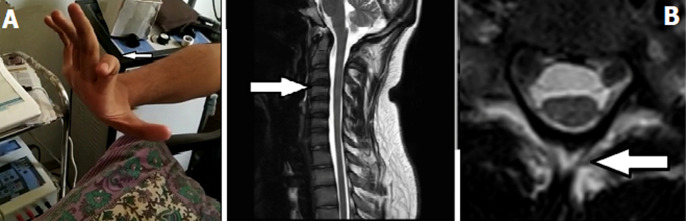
clinical as well as radiological features A) atrophy of the forearm leads to the difficulty of the movements of the hands; B) MRI findings shows unusual atrophy and changes in the cervical spine
